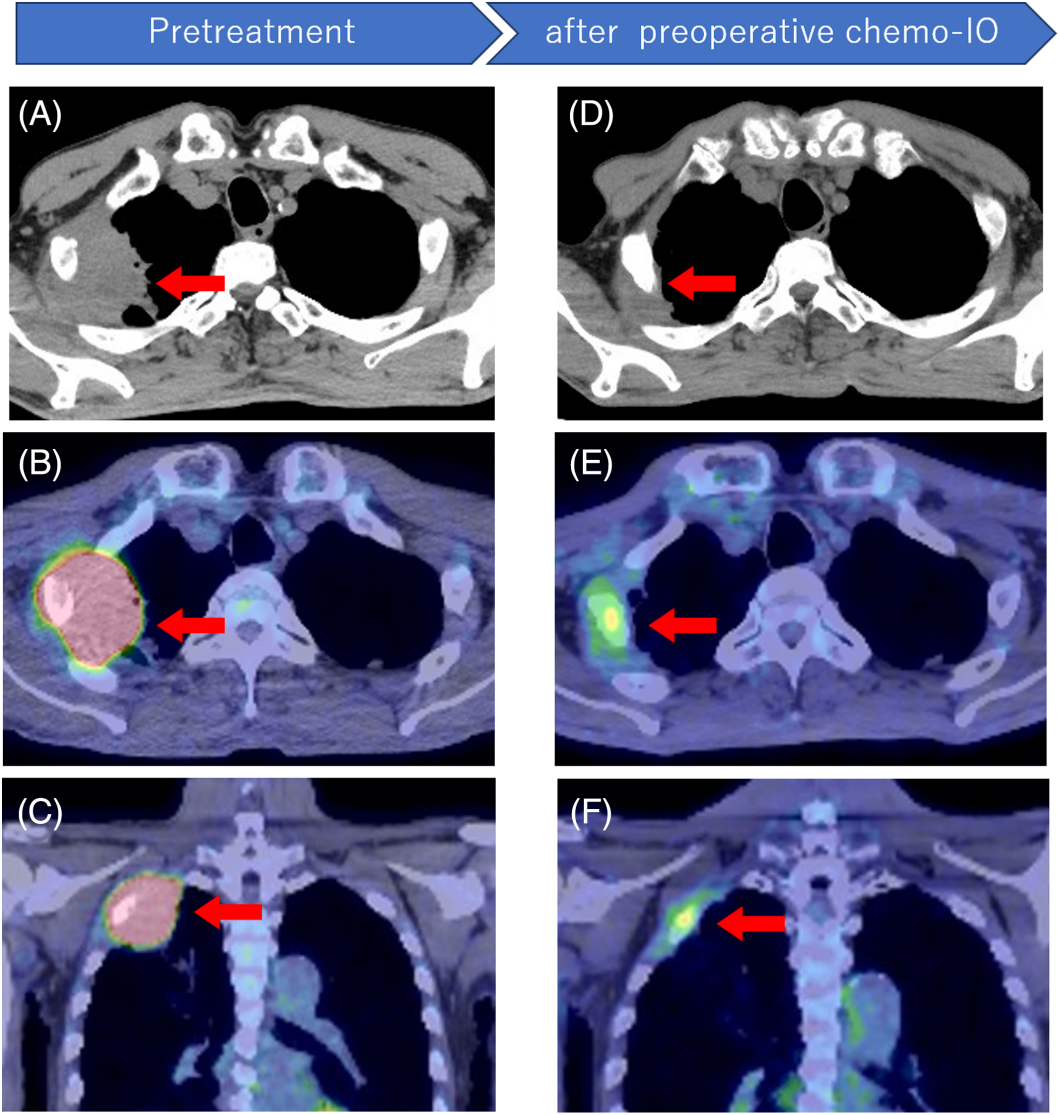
Computed tomography (CT) at first visit to our hospital in axial view (A), Positron emission tomography‐computed tomography (PET‐CT) at first visit to our hospital in axial (B) and coronal view (C), CT after neoadjuvant nivolumab and chemotherapy in axial view (D), PET‐CT after neoadjuvant chemo‐immunotherapy in axial (E) and coronal view (F).
